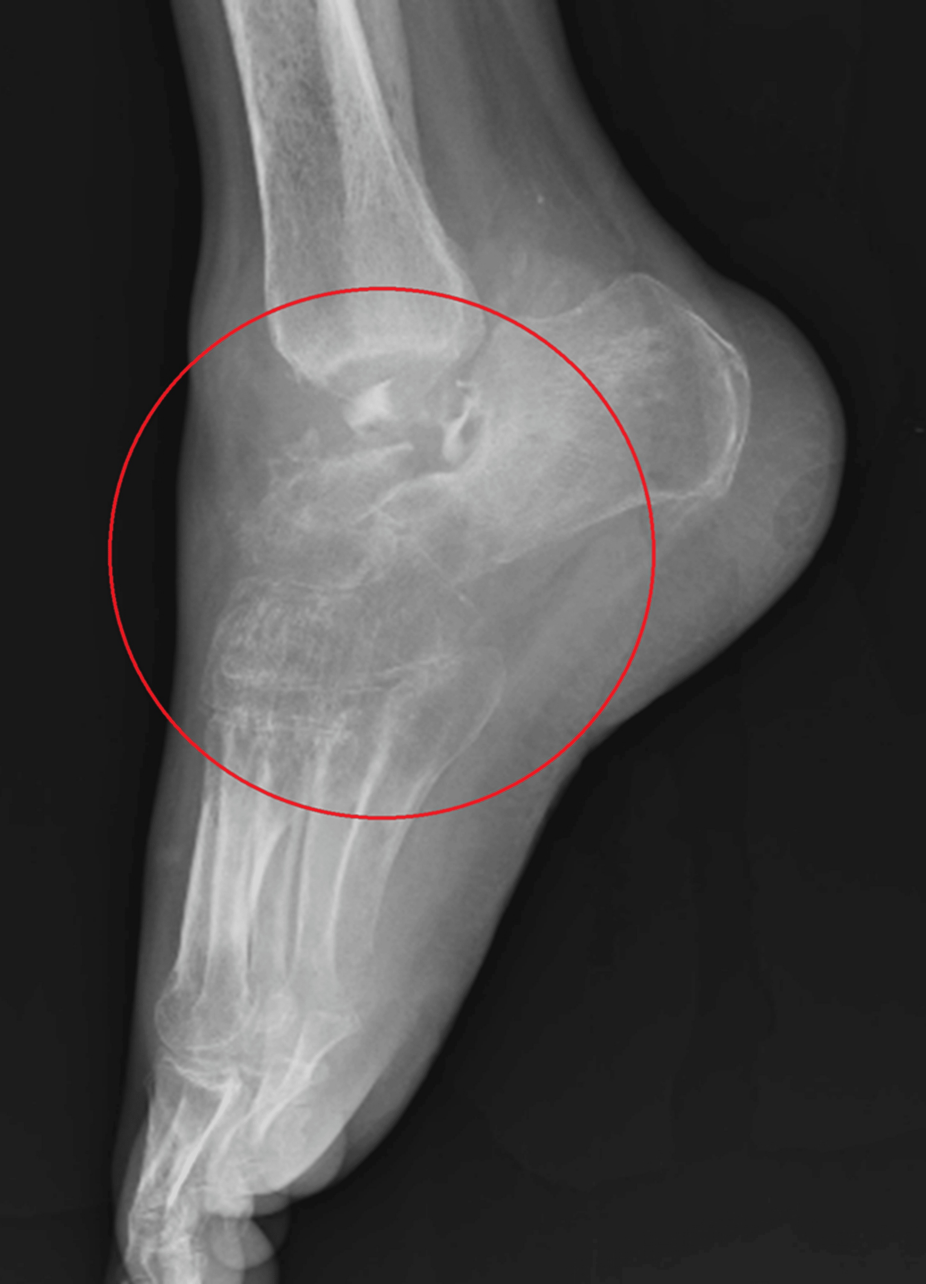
X-ray of the right ankle taken from the lateral view showing arthritic changes.
